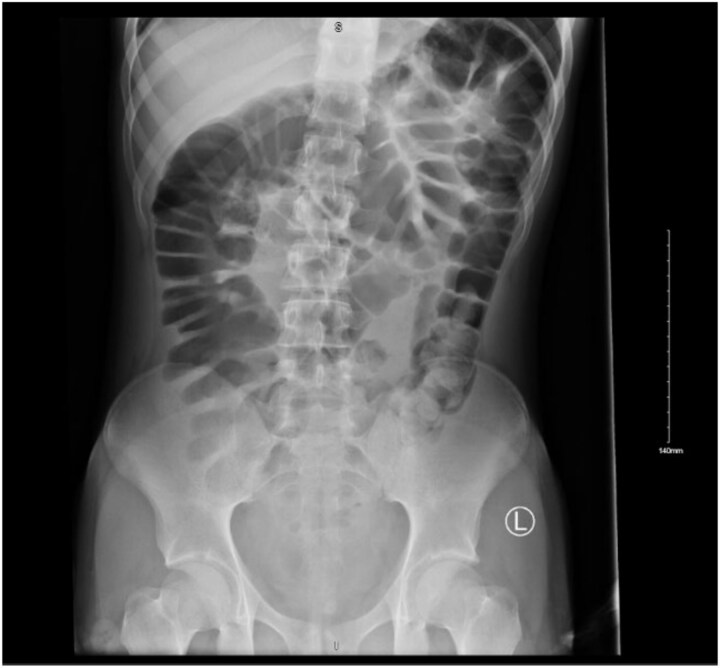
Plain abdominal radiograph demonstrating colonic distension and faecal loading of the sigmoid colon.
